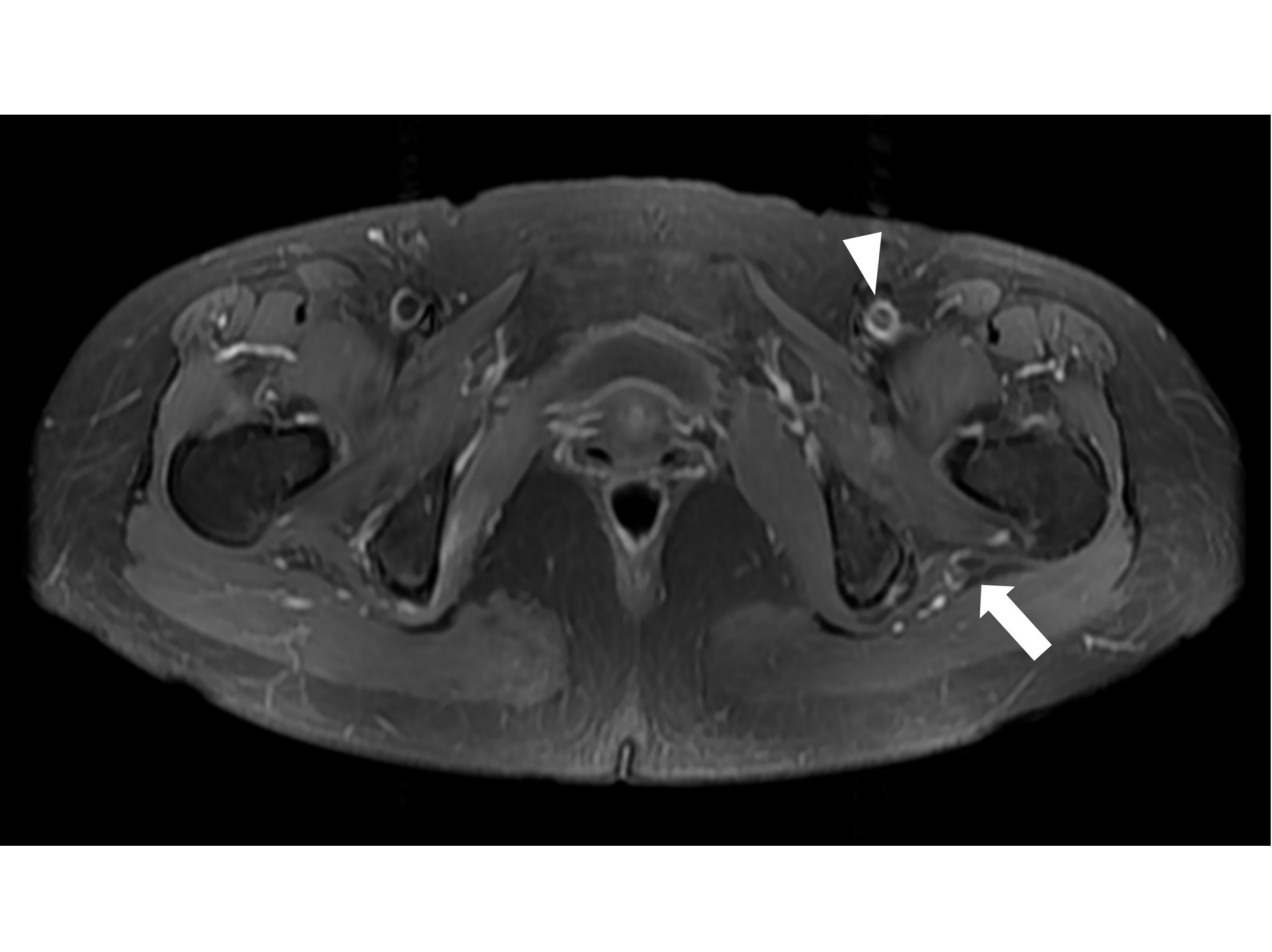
Follow-up MRI finding. Swelling and contrast enhancements of sciatic (arrow) and femoral (arrowhead) nerves were decreased after treatment.
